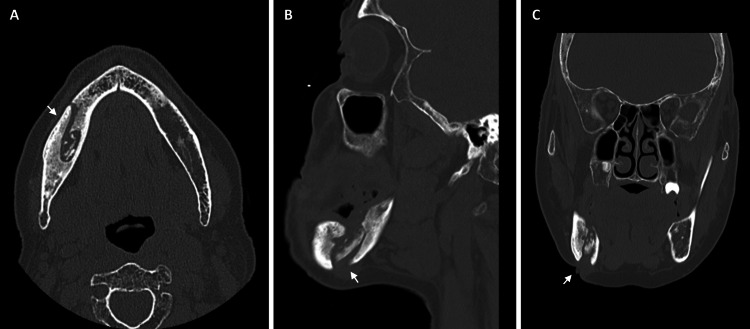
Preoperative CT bone window (A and B) Osteomyelitis of the right mandibular body with bone sequestration (white arrow). (C) Sinus tract in the right submandibular region (white arrow) and cellulitis in the right submandibular area.
